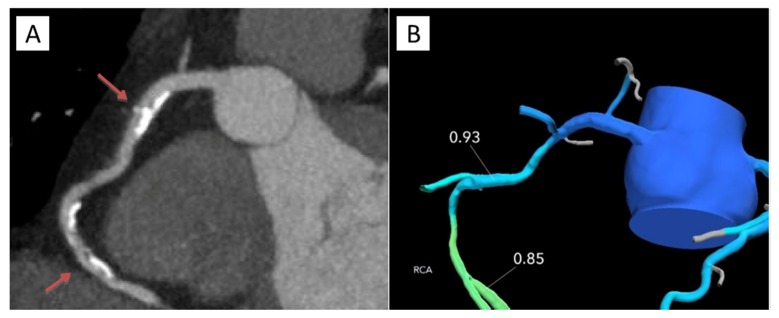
Study patient case. A 48-year-old male with a family history of coronary artery disease, dyspnea on exertion and atypical chest pain underwent coronary CTA. Multiplanar reformat of coronary CTA of the RCA (A), and FFR_CT_ (B). RCA demonstrated proximal and mid-calcified and non-calcified intermediate (50–70%) stenoses (red arrows) without evidence of lesion-specific ischemia. FFR_CT_ values distal to the proximal and mid RCA stenoses were 0.93 and 0.85, respectively. The patient safely avoided ICA and has been asymptomatic in follow-up on optimal medical therapy. FFR_CT,_ fractional flow reserve derived from coronary computed tomography angiography (CTA) datasets; RCA, right coronary artery; ICA, invasive coronary angiography.
